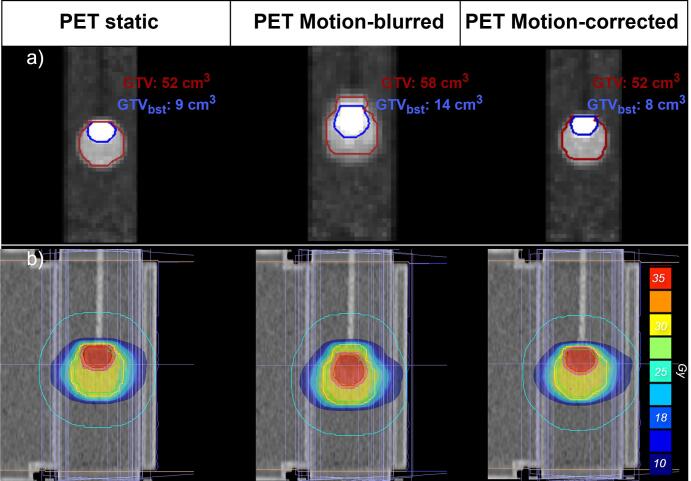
Delineation and RT-plan of the static PET, motion-blurred PET and the motion-corrected PET. a) the target delineations constructed on the PET scans with a threshold of 50% SUV_max_ for the GTV_boost_ and 30% SUV_max_ for the GTV. b) The corresponding 5 x 5 Gy + 1 Gy IMRT plans.
